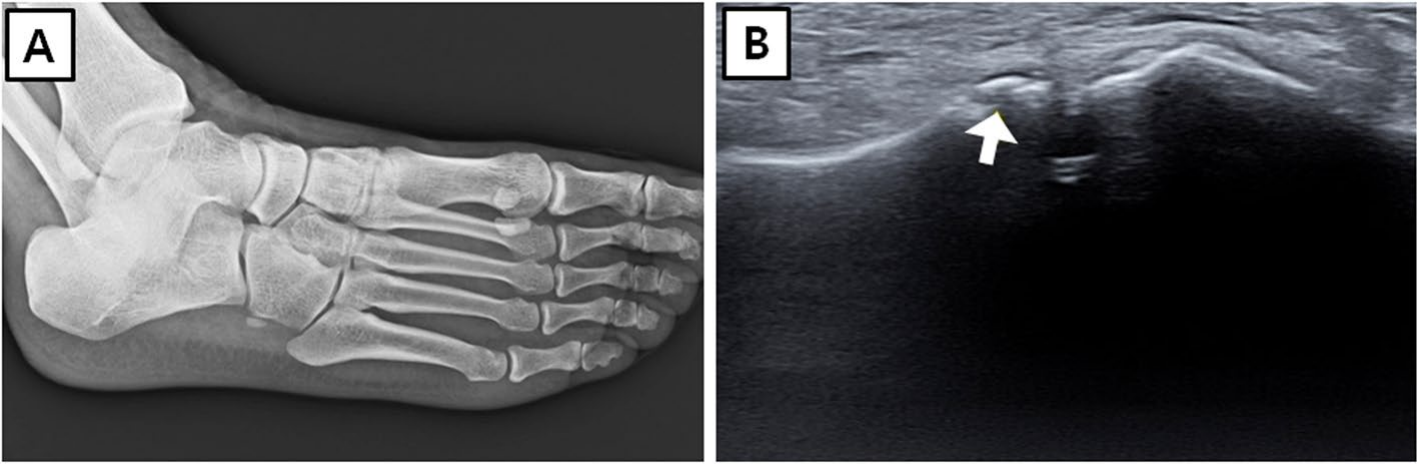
Occult fracture detection by ultrasound. A 44-year-old woman experienced an inversion sprain of right ankle. Fracture was not observed in (A) a plain X-ray, but cuboid fracture of the fourth tarsometatarsal joint was identified in (B) an ultrasound image. White arrow indicates fracture line
